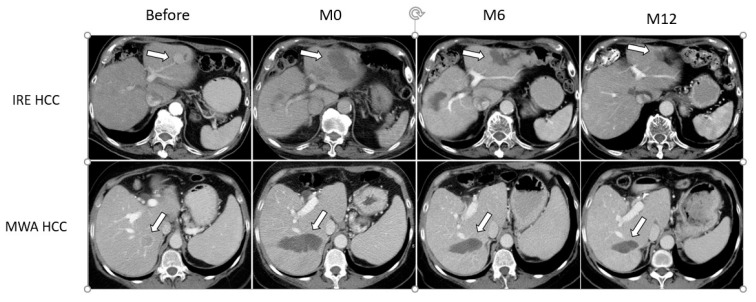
Example of ablation zone involution for HCC. Axial CT slices showing 2 HCC lesions (white arrow) in two different patients: one in left hemiliver (upper row) that was treated with IRE, and one in segments 6/7 (lower row) treated with MWA. Lesions are shown before ablation, immediately after (M0), and at 6 (M6) and 12 months (M12) follow-up. Although a perceptible difference with smaller ablation surface area is seen in patient treated with IRE, the difference between IRE and MWA did not reach statistical significance (Interaction *p* = 0.17).
